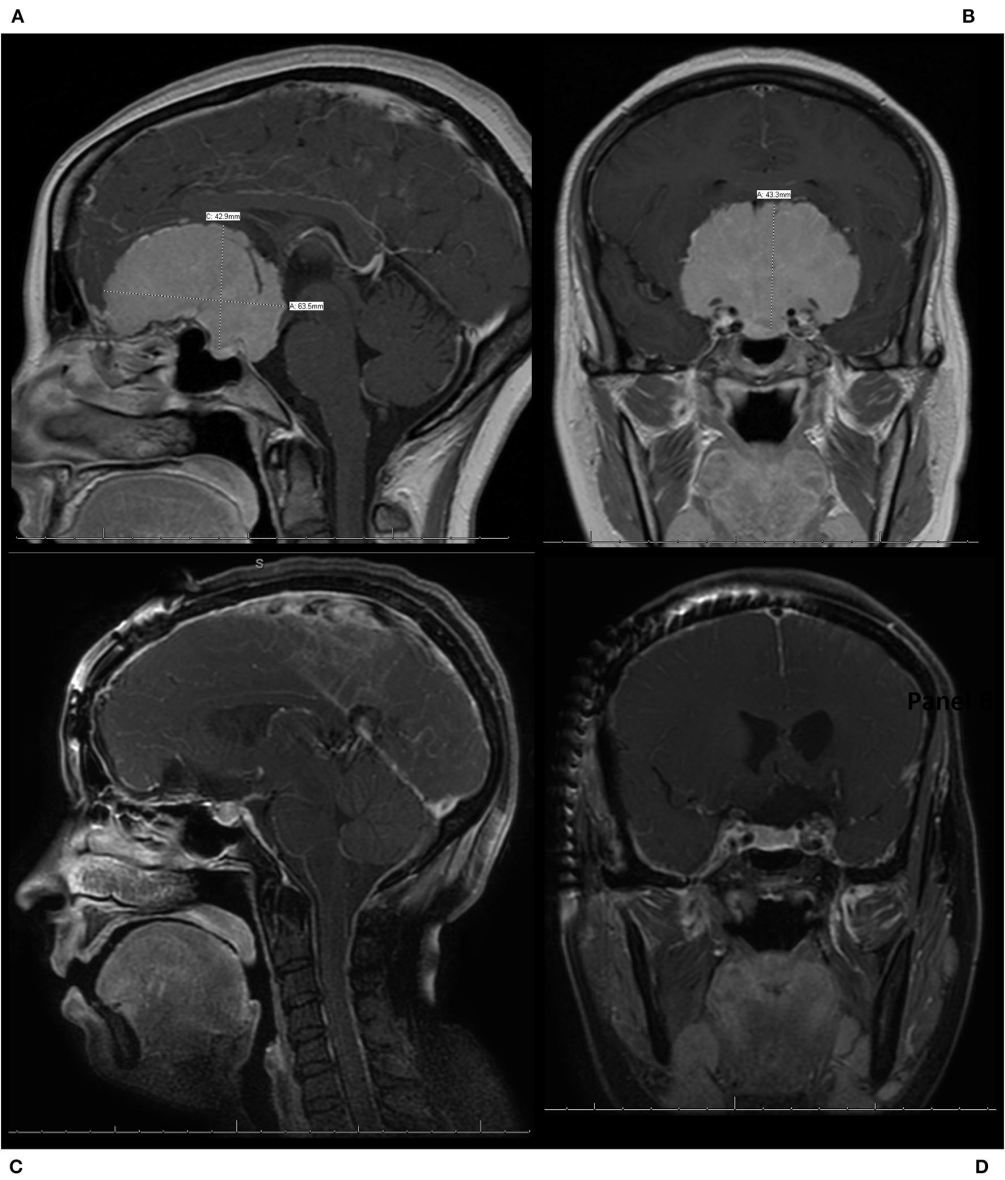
A patient who presented with progressive vision loss and anosmia was found to have a 6.4 cm olfactory groove meningioma (OGM) encasing the bilateral internal carotid artery and its branches as well as the optic nerves bilaterally. The patient underwent a modified pterional craniotomy with extension past midline to expose the superior sagittal sinus for resection of the large WHO grade I OGM. The patient had an immediate improvement in vision post-operatively, with no new neurologic deficits, and was discharged home from the hospital on post-operative day 2. (A) Pre-operative sagittal T1 MRI with contrast, demonstrating a large 6.4 cm OGM with encasement of the anterior cerebral arteries and extension in the sella seen. (B) Pre-operative coronal T1 MRI with contrast, demonstrating a large OGM with encasement of the internal carotid artery (ICA) and the middle cerebral arteries as well as the optic nerves bilaterally. (C) Post-operative sagittal T1 MRI with contrast, demonstrating resection. (D) Post-operative coronal T1 MRI with contrast, demonstrating resection with preservation of the ICAs and decompression of the optic nerves.
